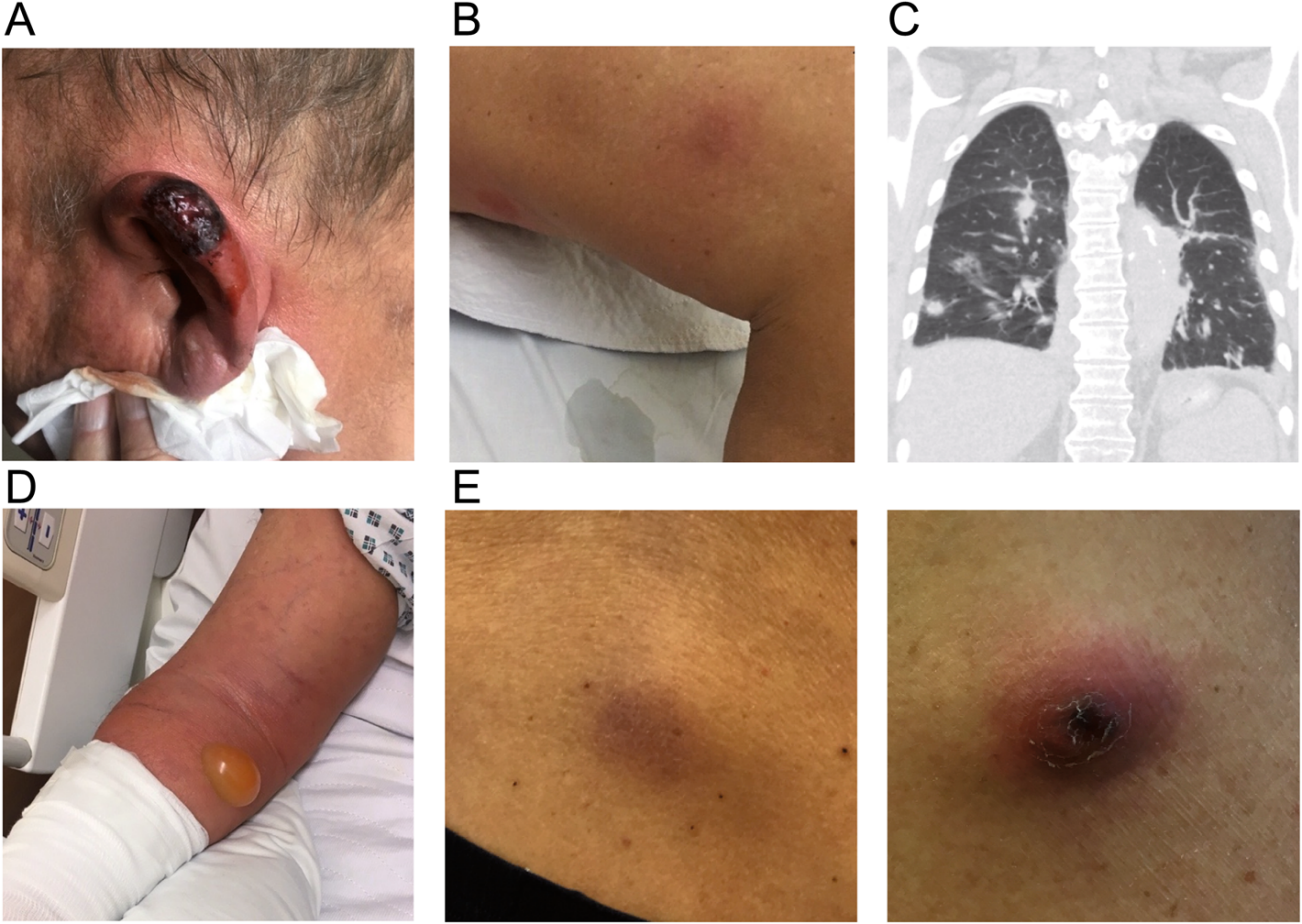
Skin and lung lesions caused by *P. aeruginosa*. a Livid discoloration of the left ear on day 0. b Erythematous skin lesions on the patient’s leg. c Chest CT scan showing bilateral pulmonary lesions. d Phlegmonous inflammation of the right arm. e Skin lesions upon secondary presentation before surgical incision
